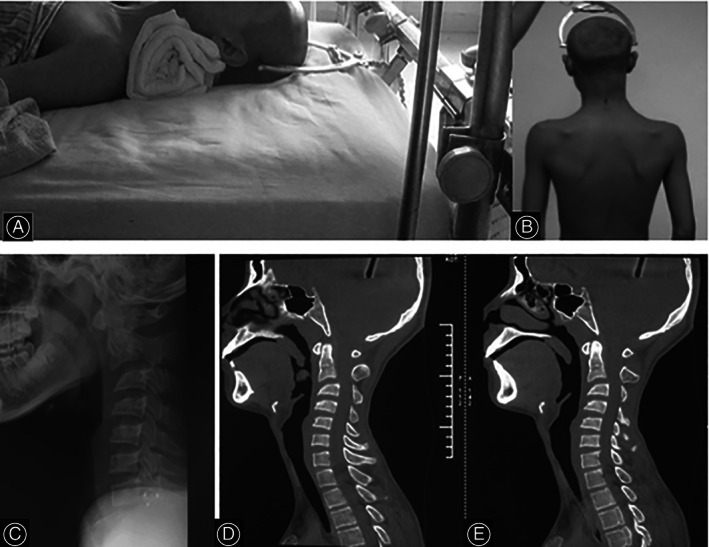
Preoperative skull traction application (A, B); lateral x‐ray (C) and sagittal CT scan (D and E) showing a significant reduction of the cervical deformity after a week intervention.
